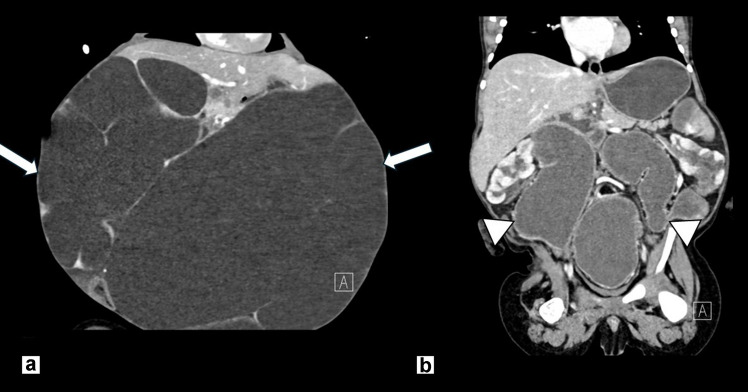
a  Coronal CT abdomen/pelvis with IV contrast image obtained in a 12-month-old boy with MMIHS and abdominal distension showing very severe bilateral hydroureteronephrosis and cortical thinning (*arrows*). (b) Coronal CT abdomen/pelvis with IV contrast image obtained in the same patient at age 3 with improved hydroureteronephrosis but persistent marked hydroureter (*arrowheads*) following ureterostomy
